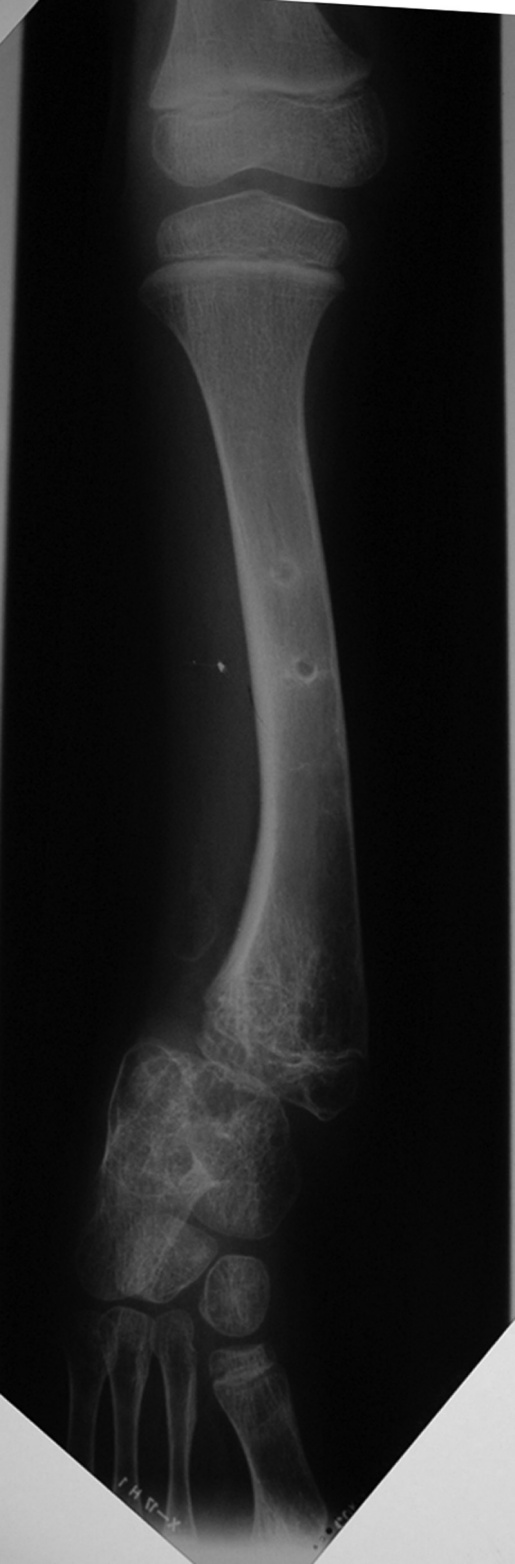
Radiographic presentation of the same patient two years after new surgical intervention. Radiograph of the same patient in the age of eleven years old and two years after new surgical intervention. The patient underwent a simultaneous closed corrective osteotomy and lengthening of the tibia. Thirteen months after completion of the consolidation phase there is a marked improvement although a distal tibia angulation of 12° still remains. Insufficient lateral buttress of the talus contributes to a valgus deformity of the ankle.
